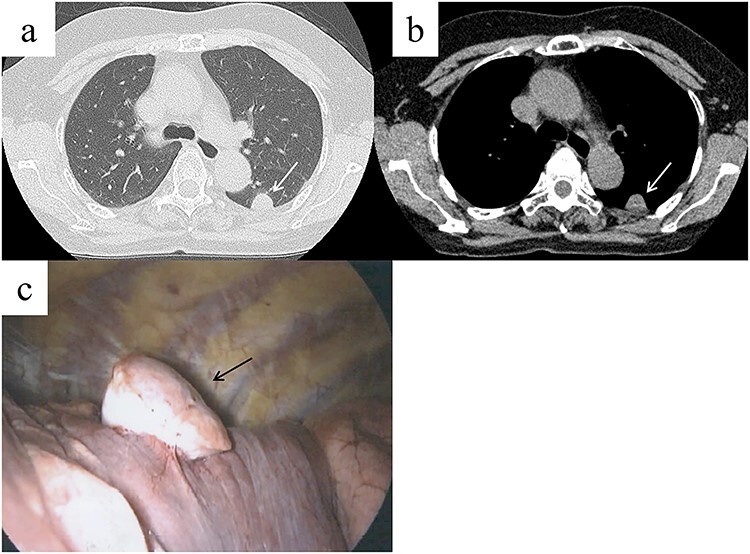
Chest computed tomography (CT) shows a well-demarcated solid nodule in the left lower lobe adjacent to the pleura (a, b, white arrow). Intraoperative findings show a stemmed tumor arising from the visceral pleura of the left lower lobe (c, black arrow).
